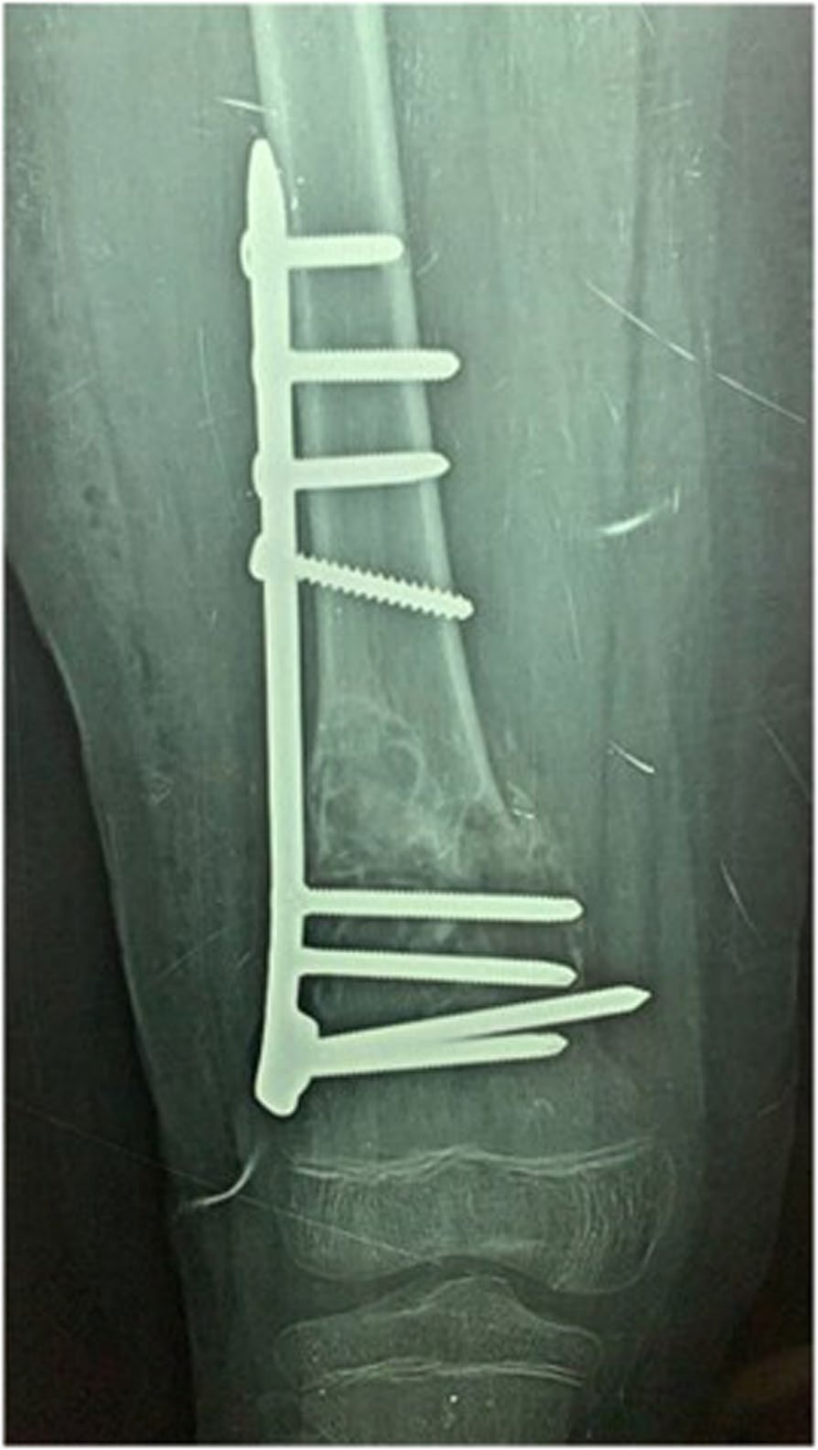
Post-operative xray after reduction and fixation of the pathological fracture
